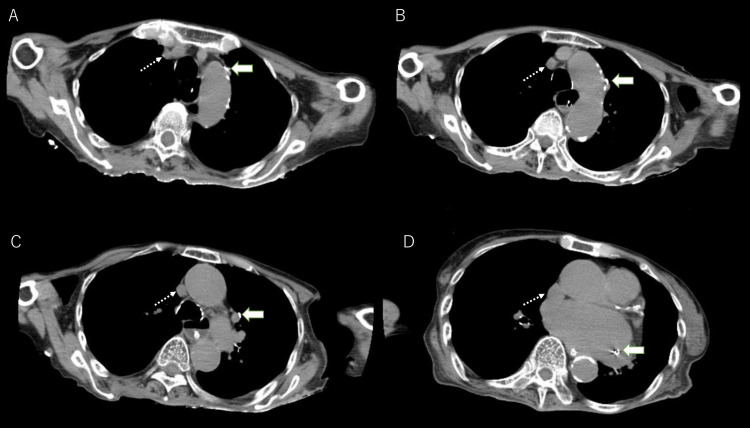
Intraoperative CT images with the guidewire inserted into the PLSVC. (A) PLSVC (solid arrow) and right superior vena cava (RSVC, dotted arrow) coexited. (B) PLSVC was extremely thin compared to RSVC. (C) There were no bridging veins between PLSVC and RSVC. (D) The PLSVC entered the CS.
